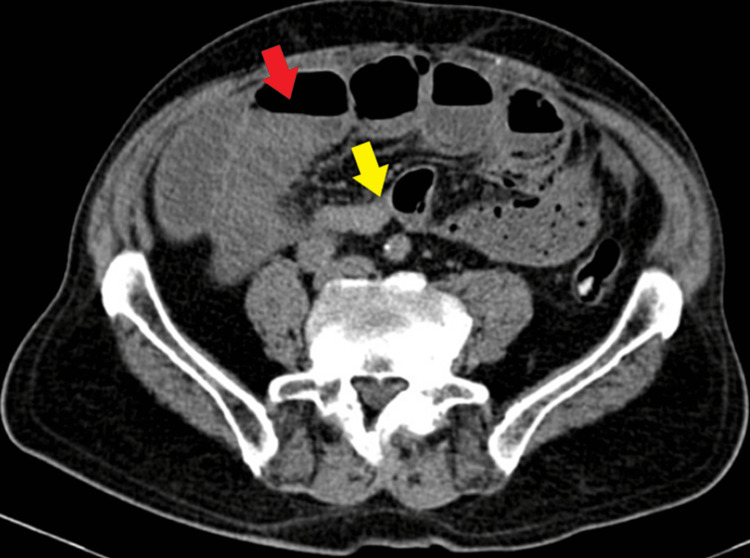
Non-contrast CT scan of the abdomen showing air fluid levels in the small bowels and a transitional zone in the distal ileum at the midline infraumbilical location. Red arrow: air-fluid levels; Yellow arrow: transition zone
